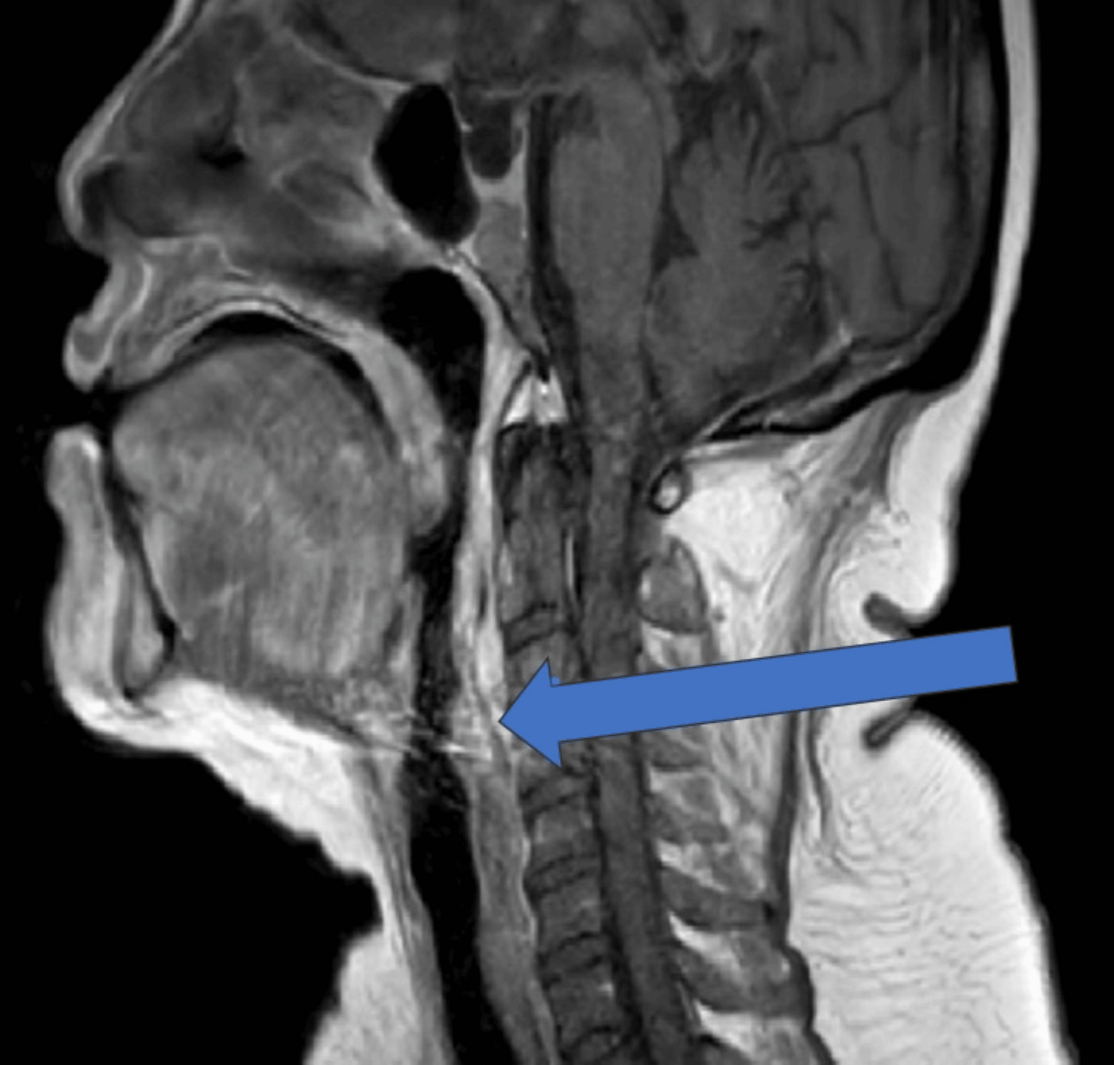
MRI of the neck showing shallow right retropharyngeal collection or abscess (improved), Day 7 of admission Improvement in retropharyngeal inflammatory changes with shallow residual collection, supporting response to conservative management.
